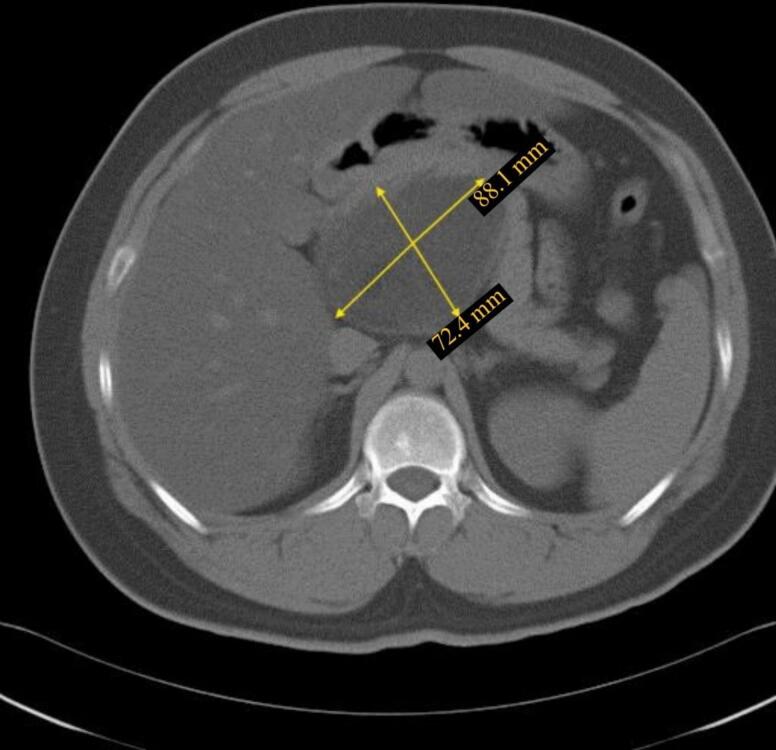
The axial CT scan without IV contrast revealed an 8.8 × 7.4 cm well-defined retroperitoneal solid hypodense mass located posterior to the head of the pancreas.
